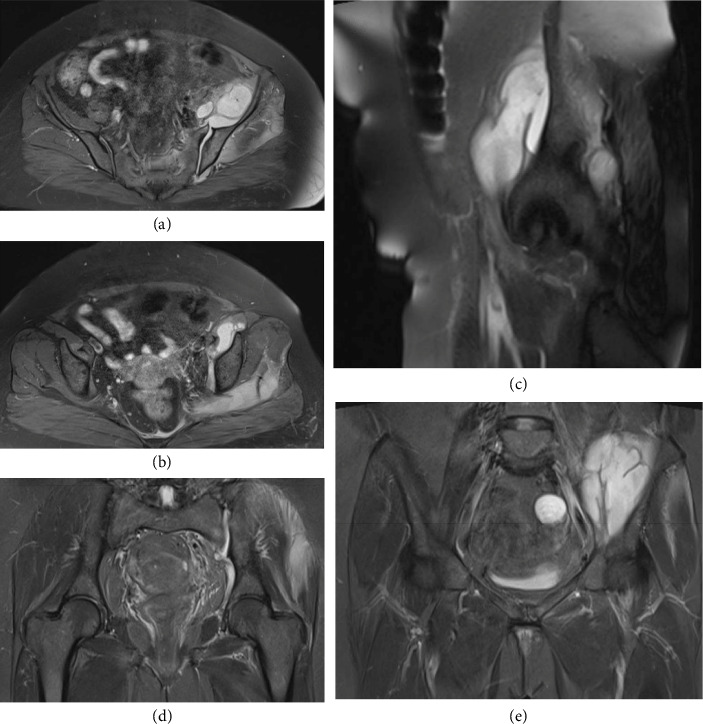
MRI study; (a-b) (axial STIR): sacroiliac joint effusion, iliacus abscess collection, and myositis involving the gluteus minimus and gluteus medius muscles. (c) Sagittal STIR: abscess within the iliacus muscle. (d) Coronal STIR: sacroiliac joint effusion and a normal hip joint. (e) Coronal STIR: iliacus abscess formation and an incidental simple cyst in the left ovary.
